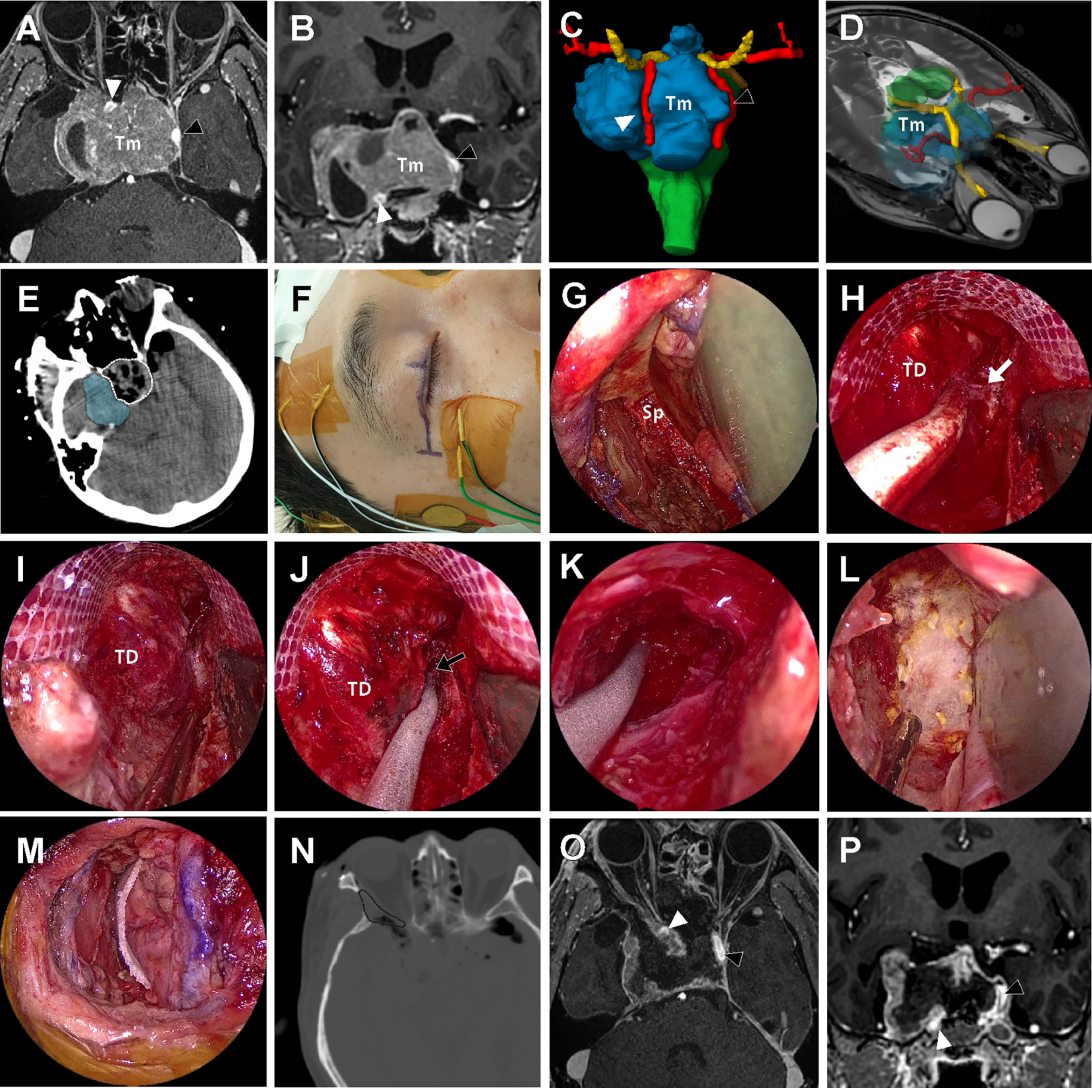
A 57-year-old male patient with history of repeated surgery and radiosurgery for an invasive pituitary adenoma. (A, B) Preoperative T1-weighted magnetic resonance imaging (MRI) with gadolinium enhancement shows a huge invasive pituitary adenoma (Tm) with right cavernous sinus (CS) invasion. The tumor pushed the right ICA (white arrow head) anteriorly and the left ICA (black arrow head) laterally. (C, D) On the three-dimensional volume rendering, the tumor (Tm, blue) was surrounded by optic apparatus (yellow) bilateral ICA (red). (E) Intraoperative computed tomography (CT) scan taken right after tumor removal under the endoscopic endonasal approach (EEA) revealed that the intrasellar tumor and suprasellar extension was successfully removed (white dotted line), but most of the tumor inside lateral compartment of CS remained (blue shadow). (F) About 3 cm-length incision along the upper eyelid crease was made for endoscopic transorbital approach (ETOA). The electrodes were placed to monitor the angle and direction of eyeball motion by triggered electrooculography and trigeminal sensory function by blink reflex. (G) After removal of lateral orbital rim, sphenoid bone (Sp) was exposed with medial retraction of orbital contents. (H) After extensive drilling of greater and lesser wing of sphenoid, the meningoorbital band (white arrow) was identified and cut to further separate the temporal dura (TD) and periorbita. (I, J) Interdural dissection was attempted but fine dissection was difficult because of postsurgical and postradiation fibrosis. Instead, window was made on the anteromedial triangle between V1 and V2. performed (black arrow). (K) As much tumor as possible in the lateral compartment of CS was removed through the anteromedial triangle. As tedious piecemeal removal of the hard tumor tissue was continued posteriorly until tumor removal cavity which was made under EEA was identified. (L, M) After meticulous bleeding control, dural and bony defect was reconstructed with synthetic surgical materials. (N) On the postoperative CT scan, the extent of bony removal for ETOA is indicated (black dotted line). (O, P) Subtotal removal of the tumor preserving bilateral ICAs (black and white arrow heads) and cranial nerves was demonstrated on the postoperative MRI.
